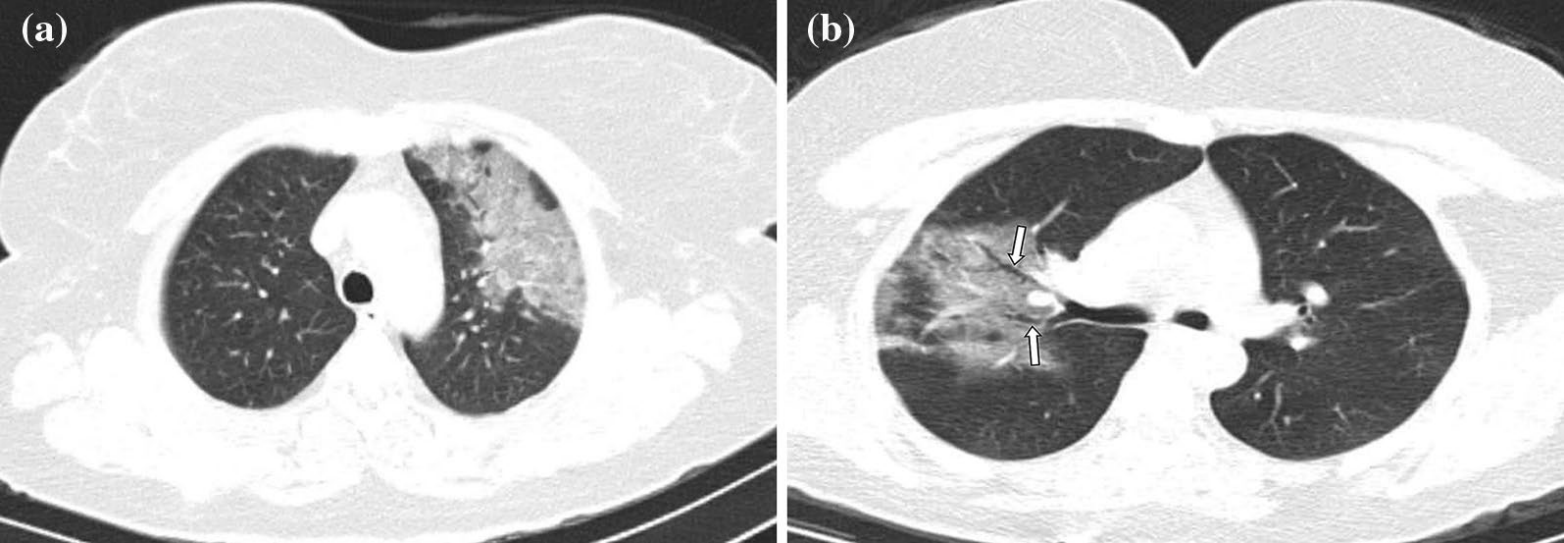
a, b Case examples defined in the classic COVID-19 for the BSTI guidance statement, and in the indeterminate category for the RSNA expert consensus statement. a An axial chest CT image of a 65-year-old female with COVID-19 shows segmental (the left upper lobe) GGOs and bronchial wall thickening. b An axial chest CT image of a 41-year-old female with COVID-19 shows segmental GGOs and vascular enlargement with bronchial wall thickening (arrows)
